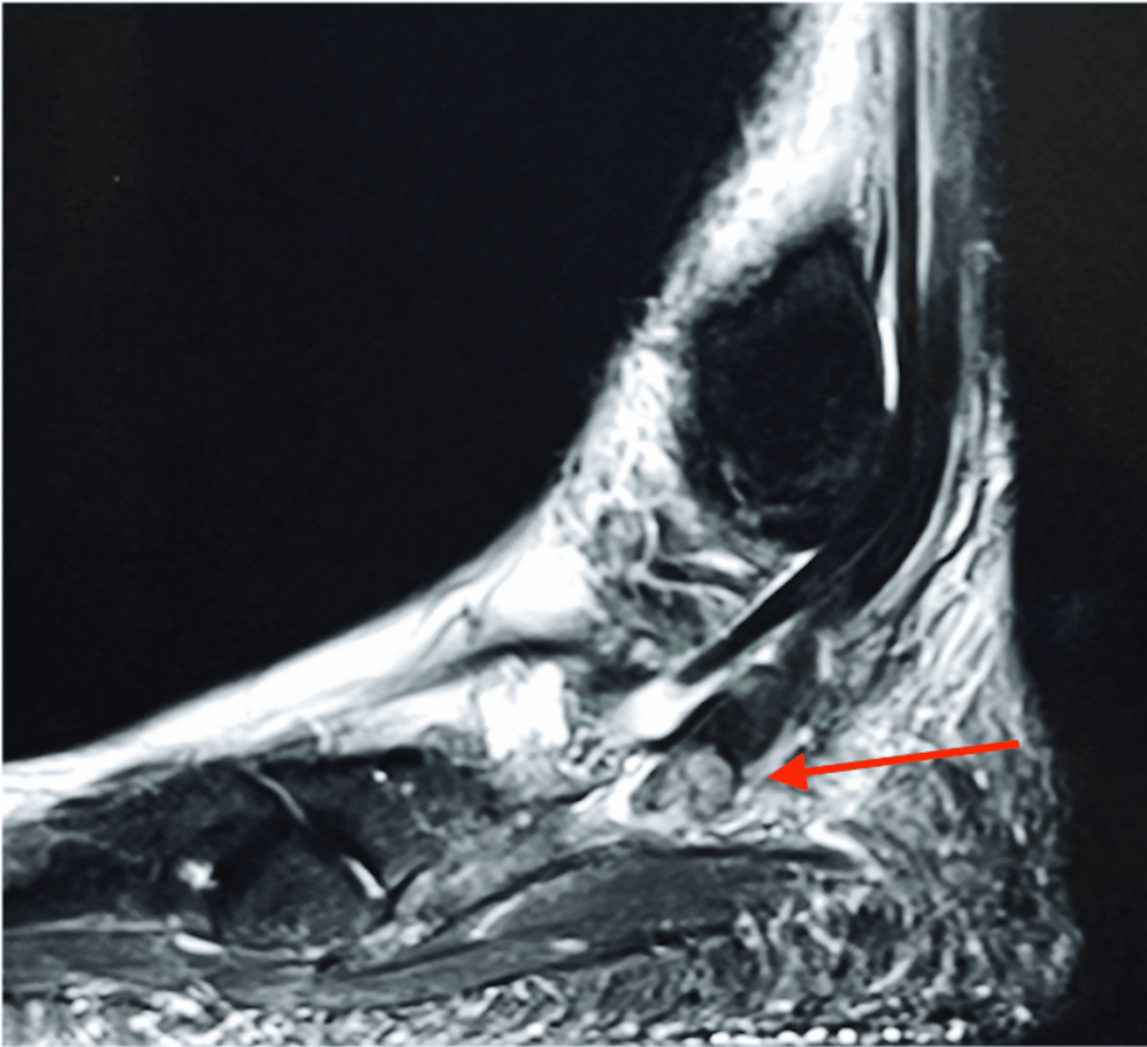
MRI sagittal image of the patient’s left ankle showing the proximal stump of the ruptured peroneal longus tendon (red arrow).
